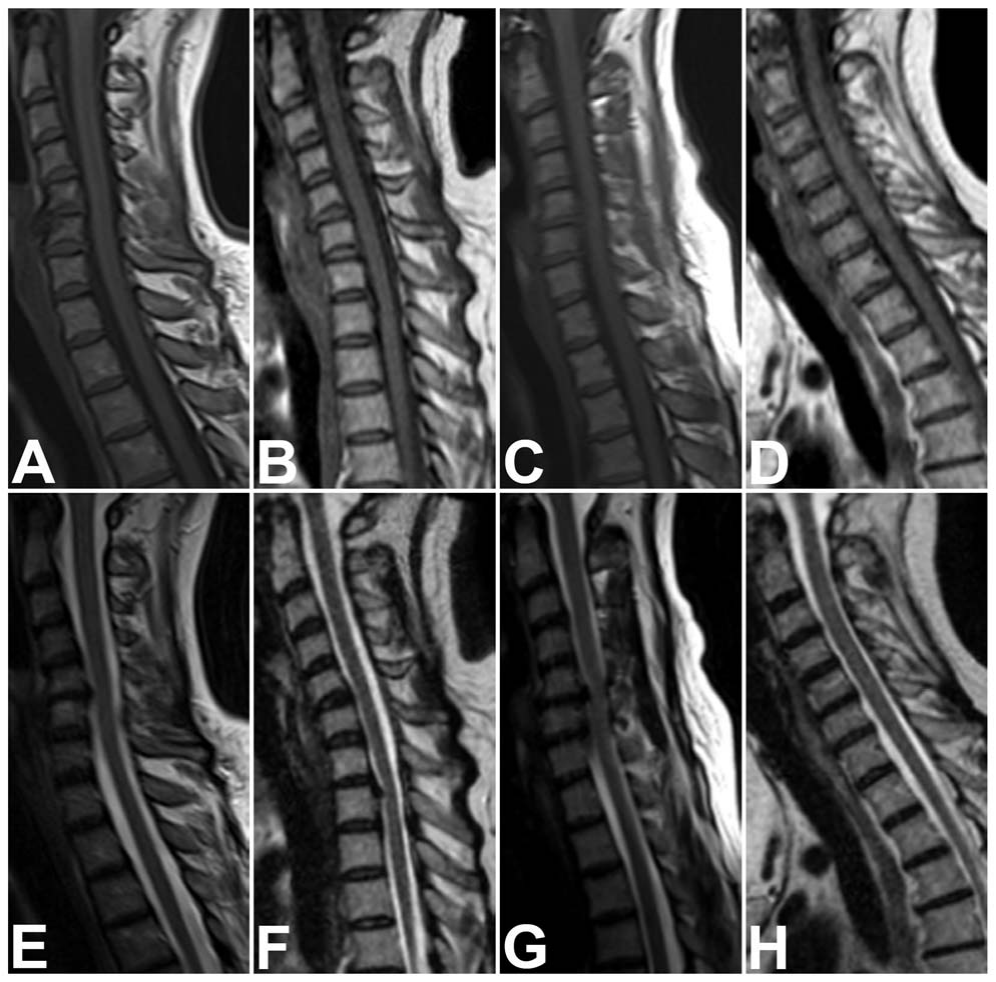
Representative Examples of Image Quality on Sagittal T1- and T2-Weighted Sequences of the Cervical Spine. Top row: sagittal T1-weighted turbo spin-echo sequences of the cervicothoracic spine of four patients, representing the range of qualitative image quality scores obtained with short-bore (A, C) and open MR imaging (B, D). Overall image quality ratings were: “optimal” for sequence A,“good” for B, “moderate” for C, and “poor” for D. No sequence was rated very poor (nondiagnostic). Bottom row: sagittal T2-weighted turbo spin-echo sequences of the cervicothoracic spine of the same four patients obtained with short-bore (E, G) and open MR imaging (F, H). Overall image quality ratings were: “good” for sequence E,“good” for F, “moderate” for G, and “good” for H. No sequence was rated very poor (nondiagnostic).
